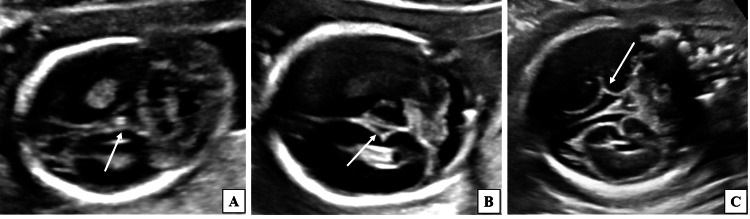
Two-dimensional ultrasound grey-scale images (coronal sections) of the fetal head demonstrating various patterns of CF at different gestational ages. (A) Fetus of 19 weeks 3 days gestational age demonstrating CF in the form of a dot pattern (long white arrow). (B) Fetus of 21 weeks 2 days gestational age demonstrating CF in the form of a V-shaped indentation (long white arrow). (C) Fetus of 25 weeks 6 days gestational age demonstrating CF in the form of a Y-shaped configuration (long white arrow). CF, calcarine fissure
